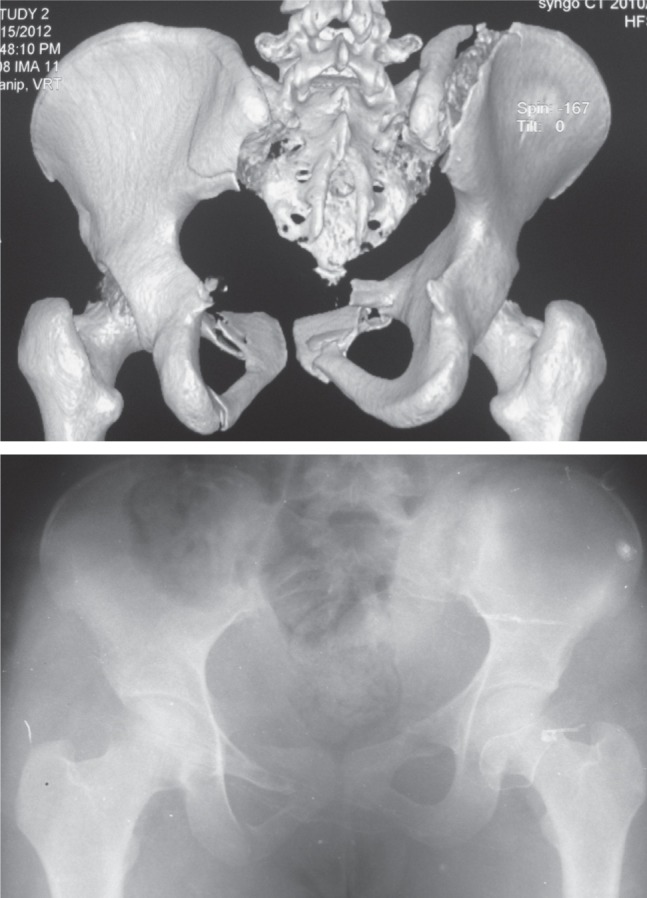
Pre-operative 3D CT scan 1B : Plain Antero-posterior radiograph respectively.
